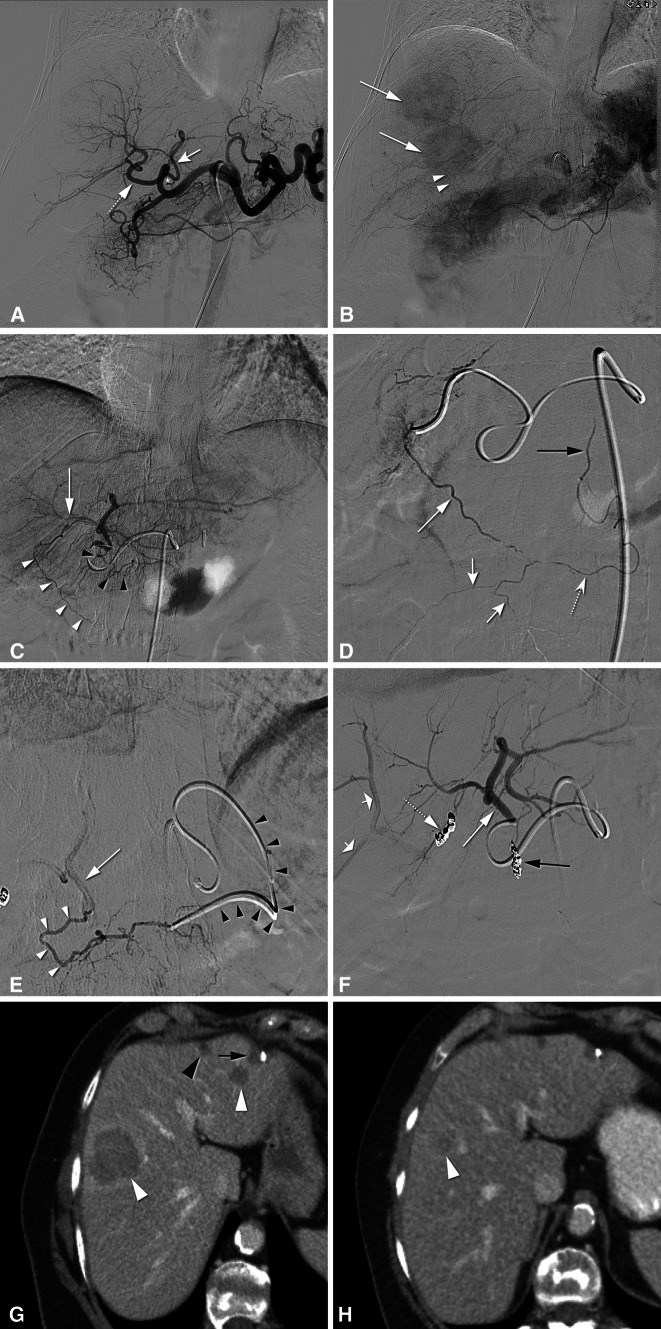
Hepatic vascular mapping in a 63-year-old female with bilateral hepatic metastases from ocular melanoma. A Angiographic images from the celiac trunk show a right gastric artery (*asterisk*) originating from the left hepatic artery (*white arrow*). B In the late arterial phase, two hypervascular metastases in the right liver lobe (*white arrows*) are seen as well as the falciform artery (*arrowheads*). A treatment plan was made to perform PHP with selective infusion of melphalan chloride into the left hepatic artery (LHA) and right hepatic artery (*dotted arrow* in A) with preemptive coiling of the right gastric (RGA) and falciform artery (FA). C Selective angiography from the LHA shows the FA (*white arrowheads*) to be originating from the segment four artery (*white arrows*). The RGA is also depicted (*black arrowheads*). D Selective angiography of the FA (*long white arrow*) shows opacification of the right internal thoracic artery (*black arrow*) through the ensiform artery (*dotted arrow*) and of anterior abdominal wall arteries (*short white arrows*). E Antegrade catheterization of the RGA was unsuccessful and therefore, retrograde catheterization was performed via the left gastric artery (LGA) using a 2.4-F microcatheter (*black arrowheads*). Angiography shows the RGA (*white arrowheads*) and LHA (*white arrow*). F Angiography of the LHA (*white arrow*) after successful coiling of the FA (*dotted arrow*) and RGA (*black arrow*) with 2-mm detachable microcoils. Some reflux of contrast is seen in right hepatic artery branches (*short white arrows*). G Axial CT image in portovenous phase before treatment demonstrates two hepatic metastases (*white arrowheads*). A third metastasis was seen in segment 4B (not shown). In the left liver lobe a cyst is seen (*black arrowhead*) as well as a hypodensity caused by previous laparoscopic excisional biopsy (*black arrow*). H CT in portovenous phase after two cycles of PHP shows marked reduction in size of the right liver lobe metastasis. The other two metastases showed a complete radiological response after treatment
